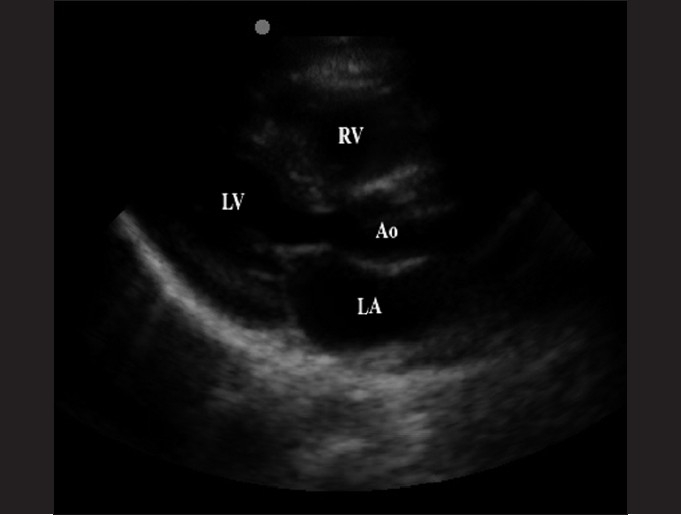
The parasternal long-axis view (LV, left ventricle; RV, right ventricle; Ao, aortic outflow tract; LA, left atrium)
